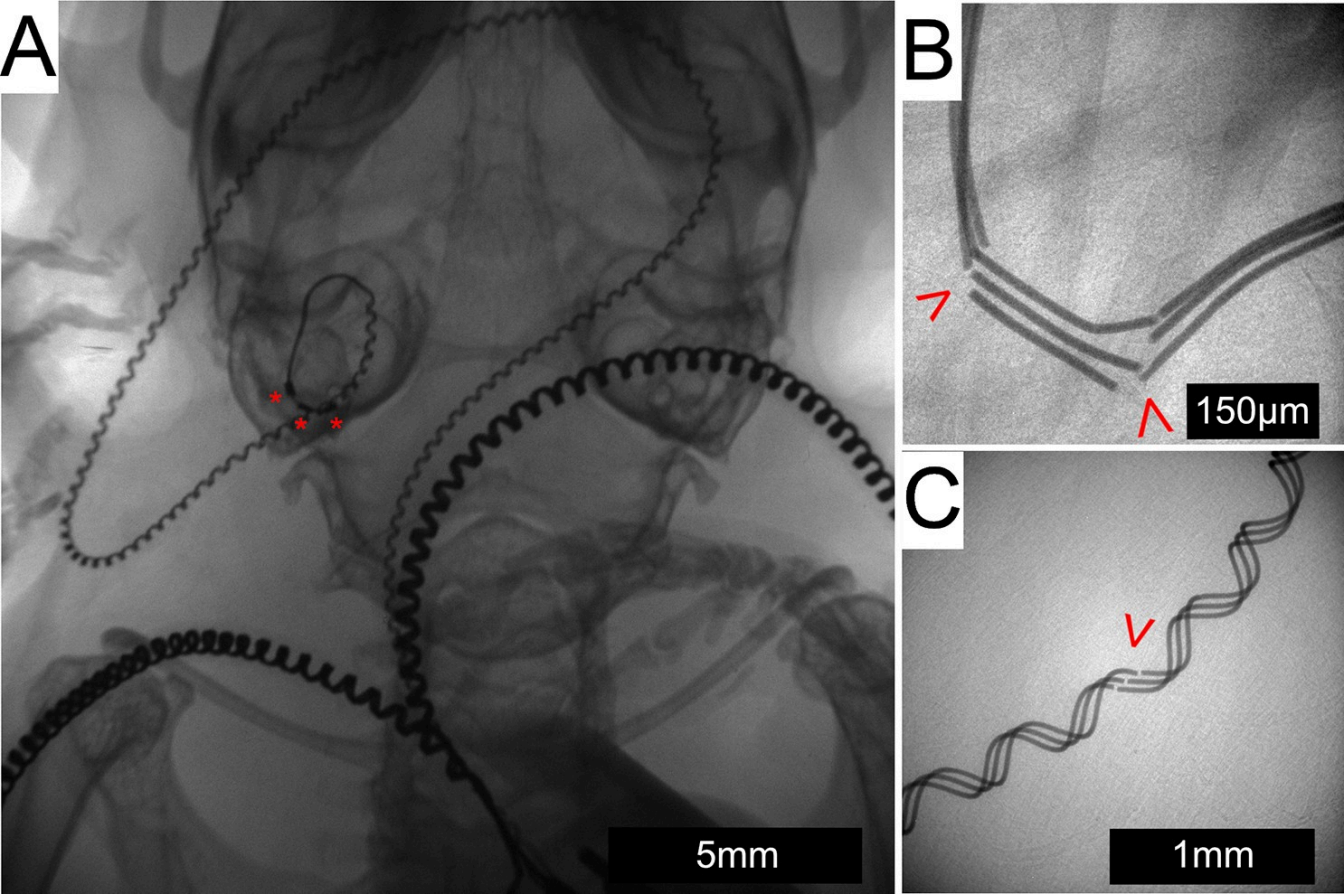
In-vivo x-ray imaging. X-ray imaging shows an intracochlear position of the array in the left cochlea of a live subject with the electrode contacts labeled by a red “*” (A). The lead wire can be seen traveling across the posterior base of the skull to meet the 2 extracochlear electrode leads as they head toward the connector located on the back (not seen in this image). Fractures (red arrowhead) occurred in both the straight (B) and helixed portions (C) of the array lead wire.
